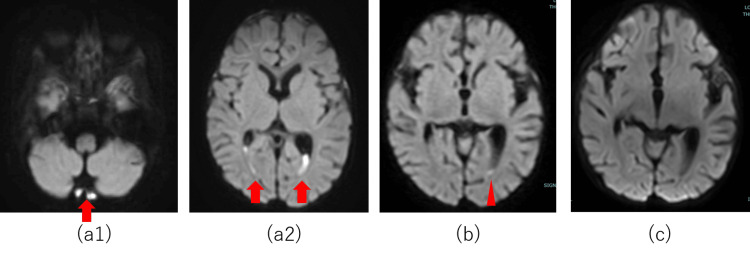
DWI findings on brain MRI On day 5, diffusion-weighted images revealed regions of hyperintensity in the posterior cranial fossa and dorsal horns of the bilateral lateral ventricles (a1 and a2). On day 12, these regions exhibited contractions (b). By day 29, these regions were completely resolved (c). DWI: diffusion-weighted imaging, MRI: magnetic resonance imaging
